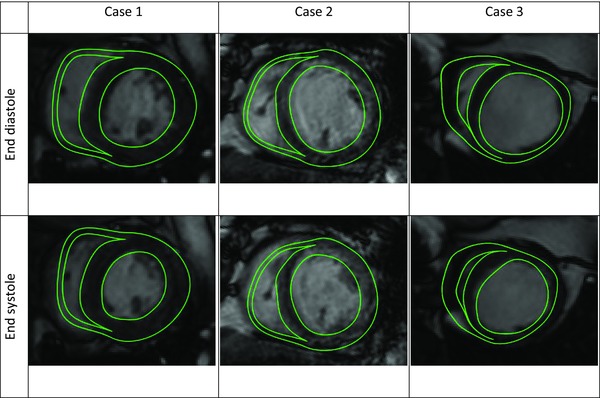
The motion of the cardiac models during the cardiac cycle was validated against cine MR images.
